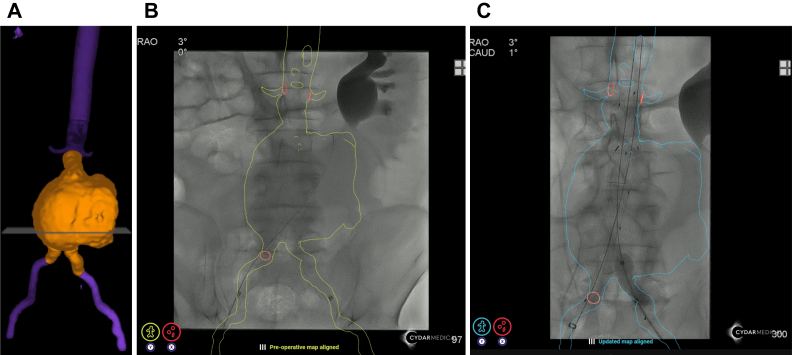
(A) Three-dimensional aneurysm anatomy. Displayed is the reconstructed three-dimensional representation by Cydar EV Maps; this tool also enables automated volume measurement of the aneurysm. The aneurysm sack is displayed in *orange*, and the suprarenal aorta and iliac vessels are displayed in *purple*. (B) Cydar EV Maps vessel overlay. Displayed is the automated rigid vessel overlay during surgery prior to angiography. Computer vision continuously watches the X-ray images, and after identifying two or more vertebrae, an automated overlay appears on the screen. An indication of the outer lines of the aneurysm is in *yellow*, and the ostia of the aortic branch vessels is in *red*. (C) Cydar EV Maps vessel overlay. The physician-adjusted vessel overlay after angiography is shown. It is defined while having the C-arm in the position with a perpendicular view on the orifice of the lowest renal artery. An indication of the outer lines of the aneurysm is displayed in *blue*, and the ostia of the aortic branch vessels is shown in *red*. Note that the level of the renal orifices is slightly different from the automated overlay due to the adjustments. This picture is after successful exclusion of the aneurysm, ballooning the endovascular aneurysm repair (EVAR) device.
